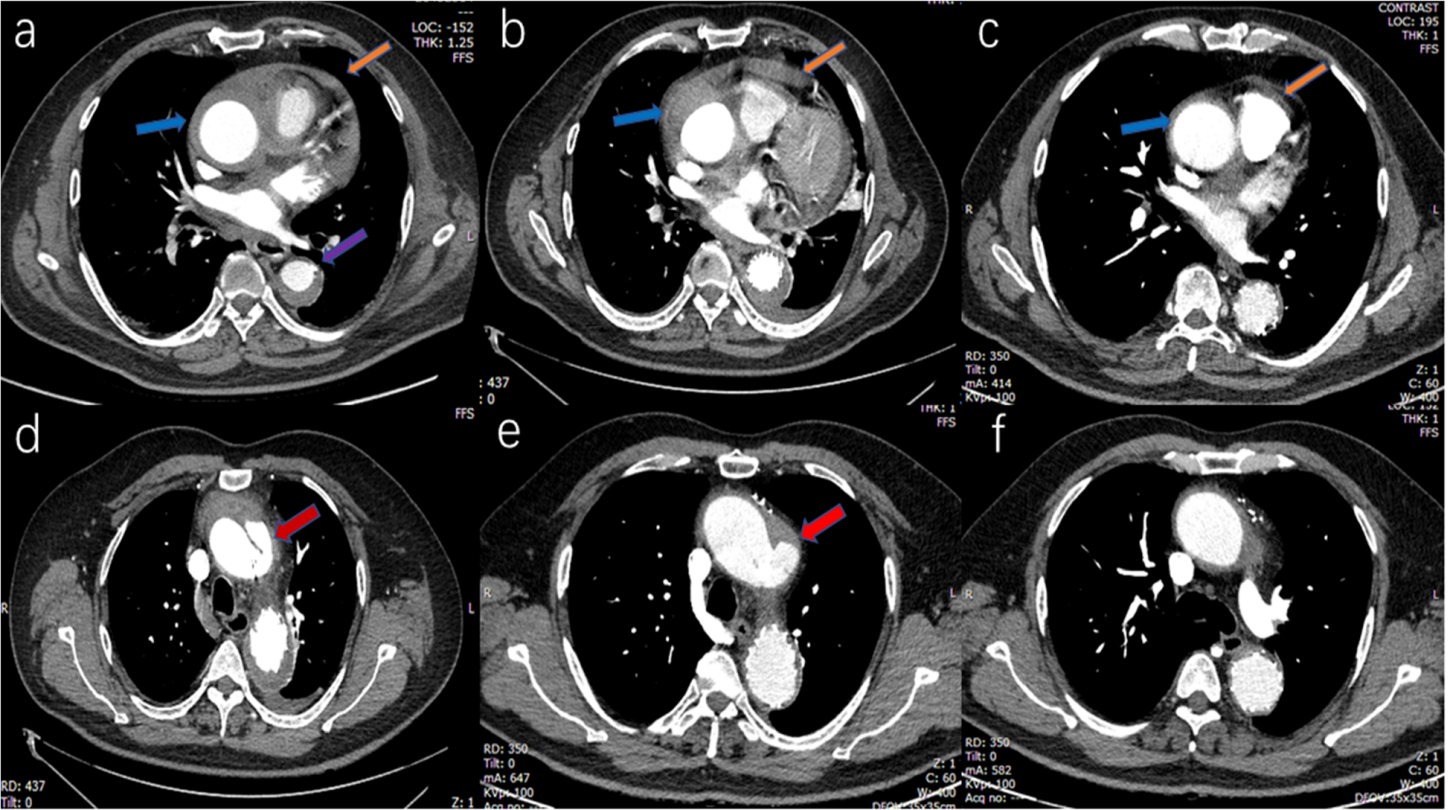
The changes on computed tomography angiography between admission and after thoracic endovascular aortic repair (TEVAR). a On admission: a penetrating atherosclerotic ulcer (PAU, purple arrow) located in descending aorta, the ascending aortic intramural hematoma (blue arrow) and pericardial effusion (brown arrow). b 2 weeks after TEVAR: PAU was excluded but the maximal ascending aortic diameter (MAAD) and the maximal ascending aortic hematoma thickness (MAHT, blue arrow) increased, and the pericardial effusion (brown arrow) and pleural effusion progressed. c 3 months after TEVAR: the MAAD decreased with the MAHT (blue arrow), the pericardial effusion (brown arrow) and the pleural effusion nearly disappearing. d 2 weeks after TEVAR: an aortic arch dissection (red arrow) distal to ascending aorta occurred. e 3 months after TEVAR: the aortic arch dissection (red arrow) stabilized. f 3 months after TEVAR: a dissection was confined within the aortic arch
